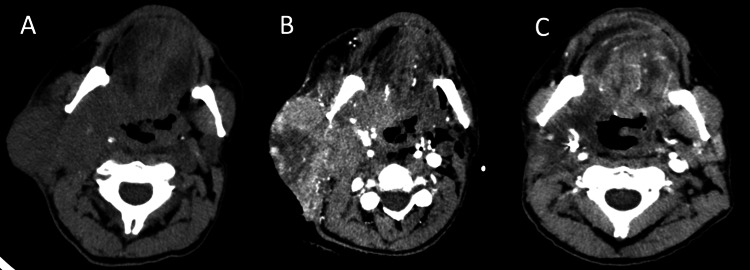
CT of the neck without contrast, 05/2021 (A); CT of the neck with contrast, 06/2021 (B); CT of the neck with contrast after embolization with chemoradiotherapy, 11/2021 (C)
